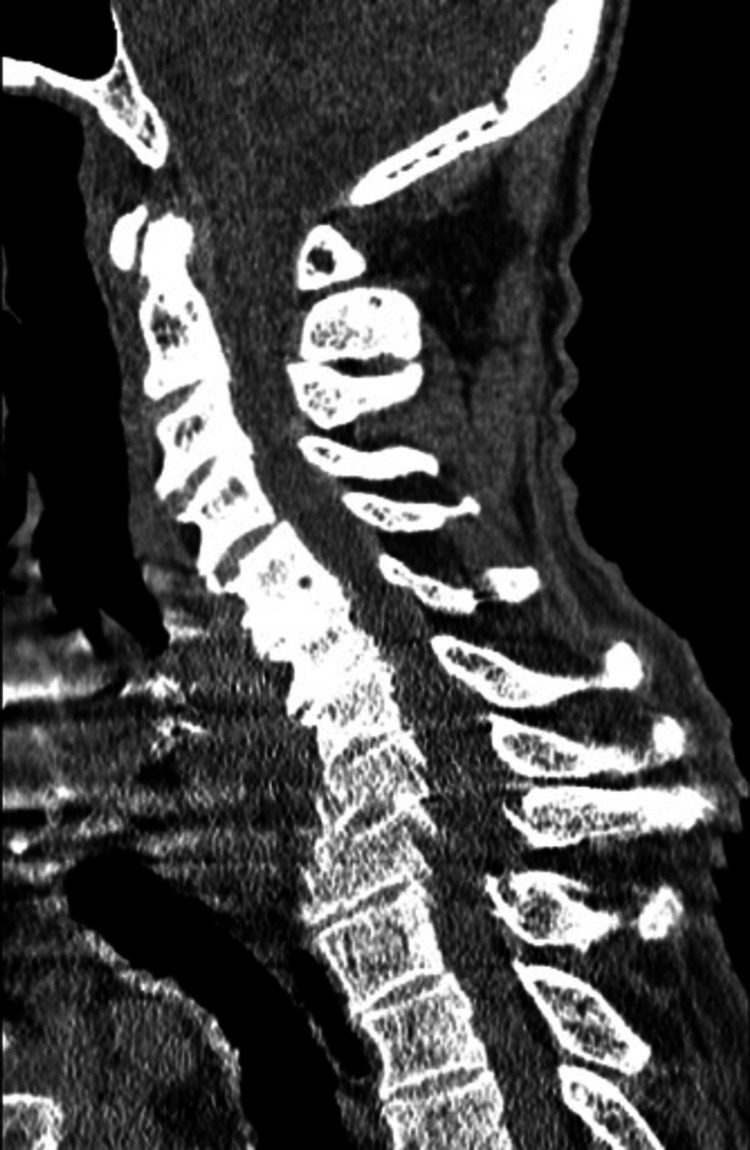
Normal cervical spine computerized tomography (CT) scan
